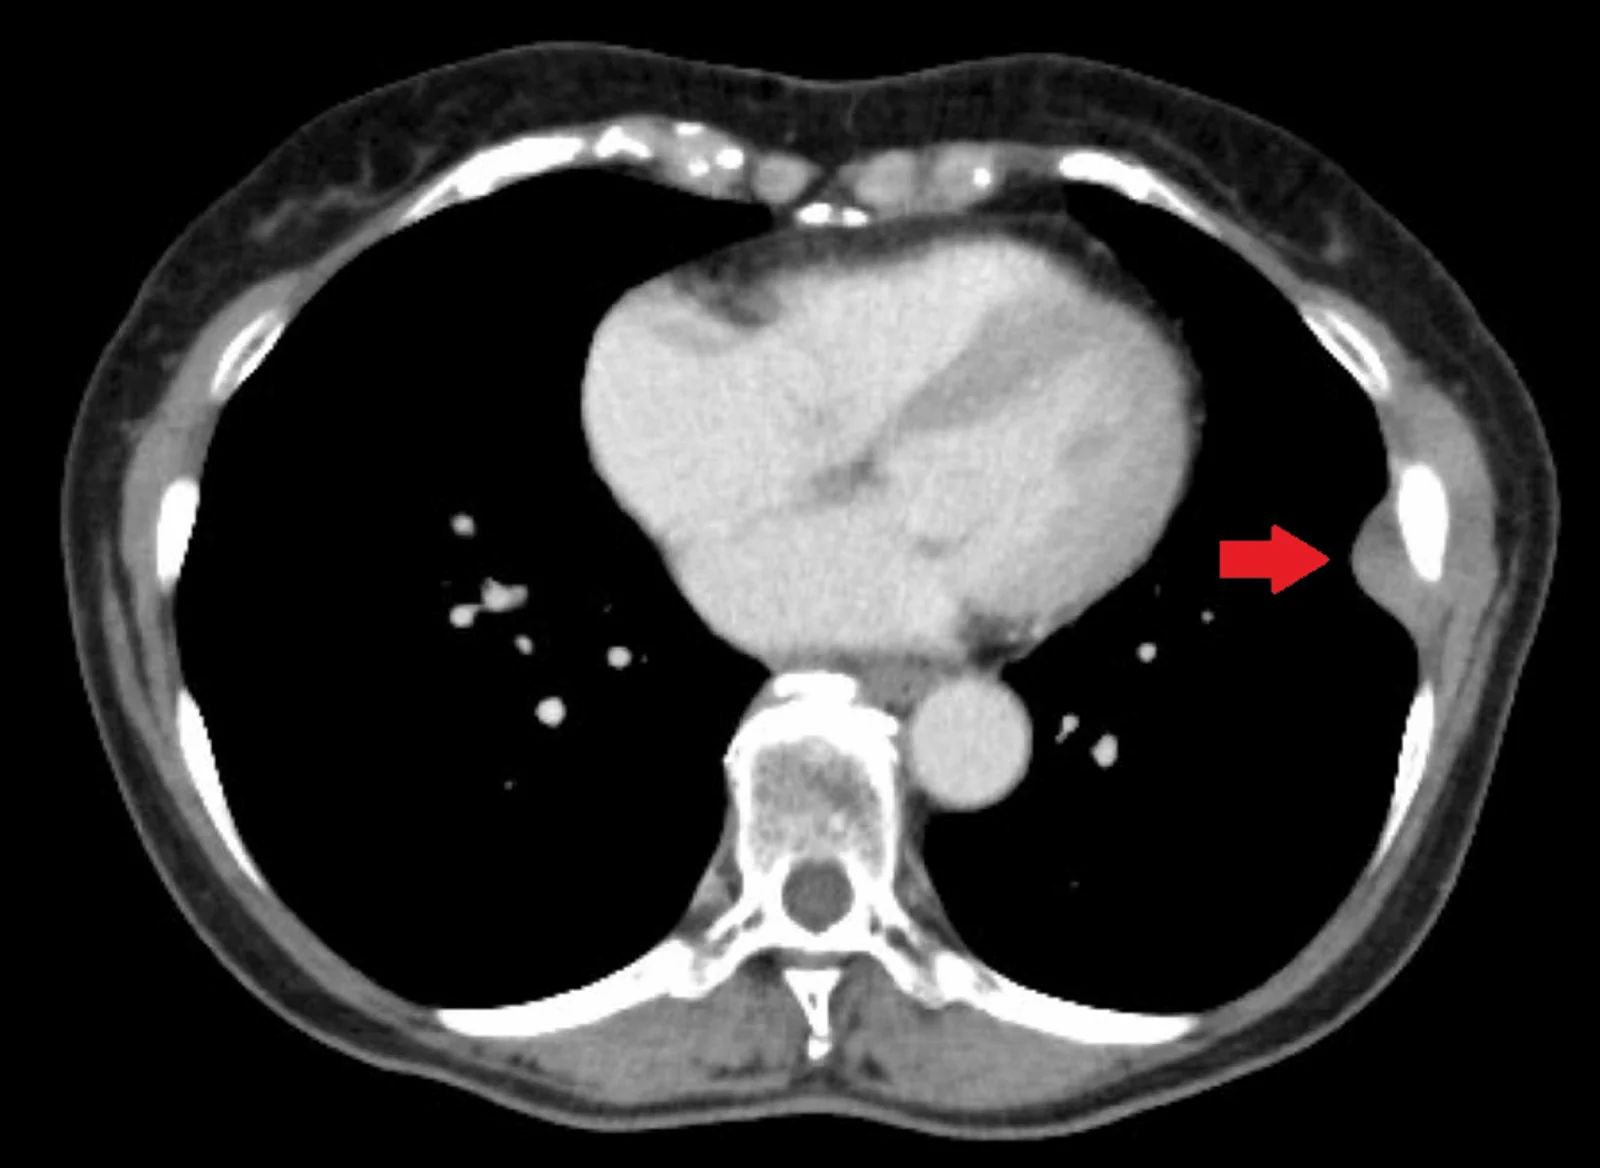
CECT CECT revealed a 30 mm × 10 mm chest wall mass located just below the left seventh rib. CECT: contrast-enhanced computed tomography
